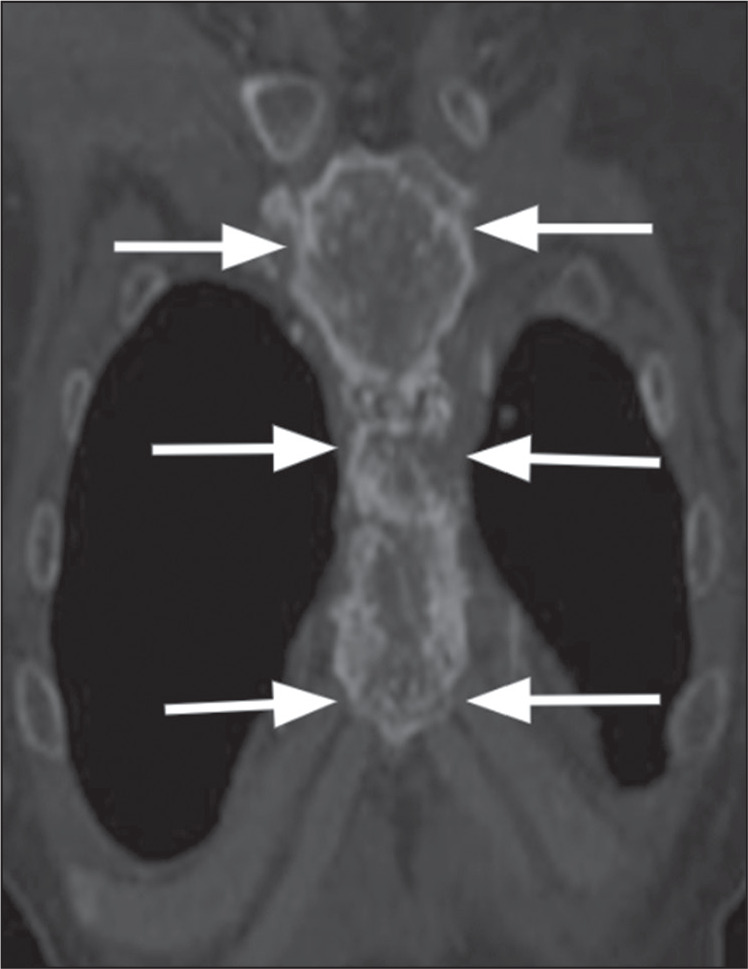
Hemangioendothelioma. Reconstruction of a coronal CT scan of the chest
